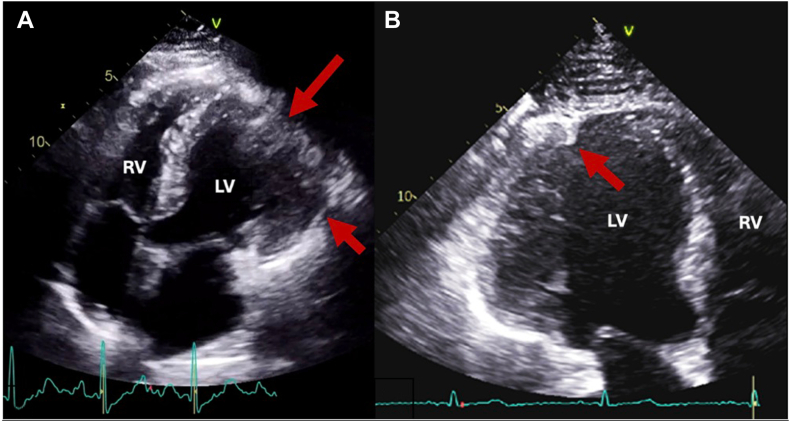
(A) Index hospitalization two-dimensional (2D) TTE, apical four-chamber view (left ventricle [LV] on *right*), demonstrates a thickened and poorly delineated anterolateral wall (*arrows*) in systole. (B) Repeat 2D TTE, zoomed-in apical four-chamber view (LV on *left*), demonstrates bulging of the thickened anterolateral ventricular wall beyond an area of constriction, where the myocardium appeared pinched. *RV*, Right ventricle.
